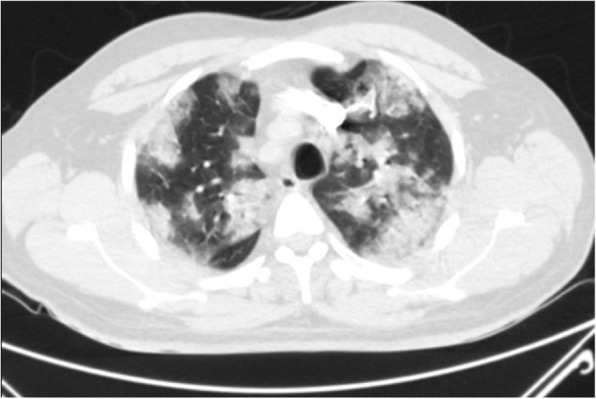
Diffuse alveolar damage: thin section CT shows patchy areas of GGO and consolidation in the lung periphery in upper lobes
